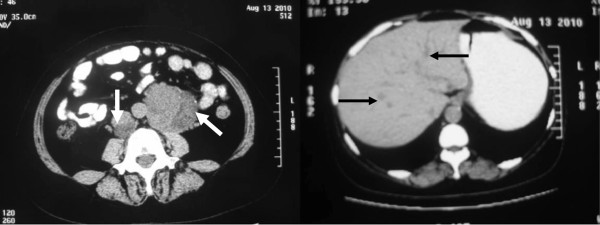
Abdominal CT scan showing two masses (white arrows) beside abdominal aorta, multiple intrahepatic hypodense nodules (black arrows) and retroperitoneal lymph nodes enlargement.
